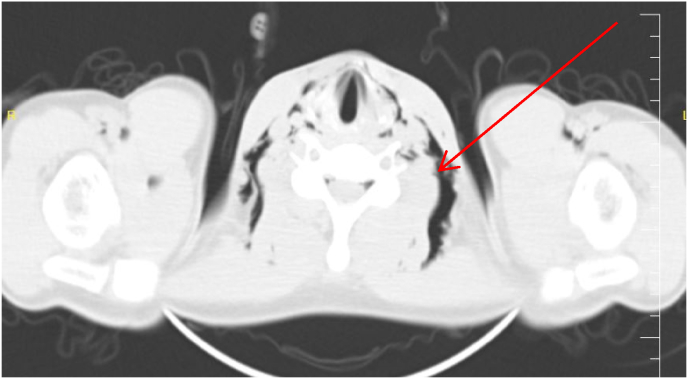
CT scan showing air collection in the bilateral neck area (arrow) indicating subcutaneous emphysema.
